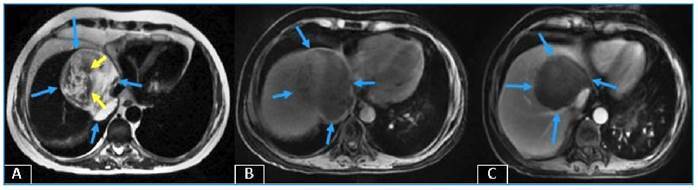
MRI showing (A) heterogeneous (containing hypointense areas: yellow arrows) hyperintense lesion on T2-weighted image (blue arrows), (B) hypointense on T1-weighted image (blue arrows), and (C) no contrast enhancement on post-contrast T1-weighted image (blue arrows).
